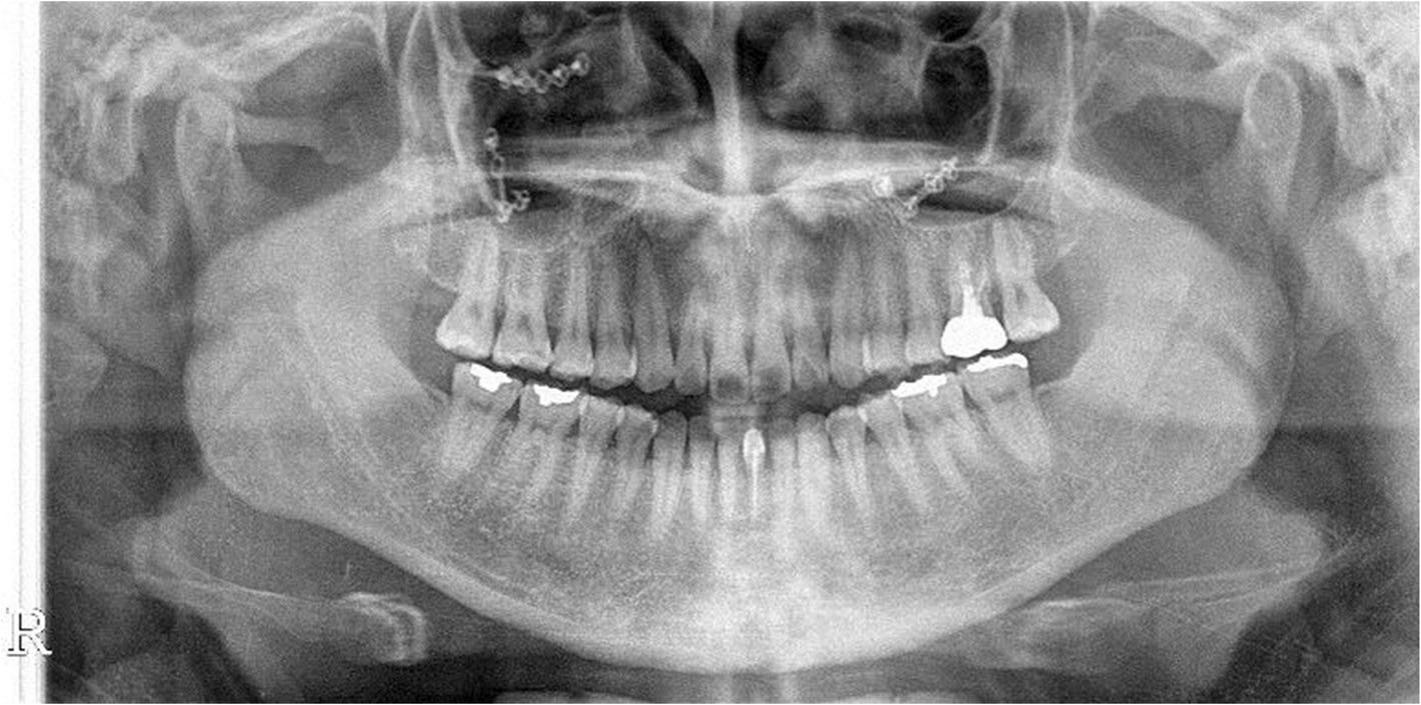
Preoperative panoramic radiograph showing malunioned segments both at the maxilla and severe malocclusion
